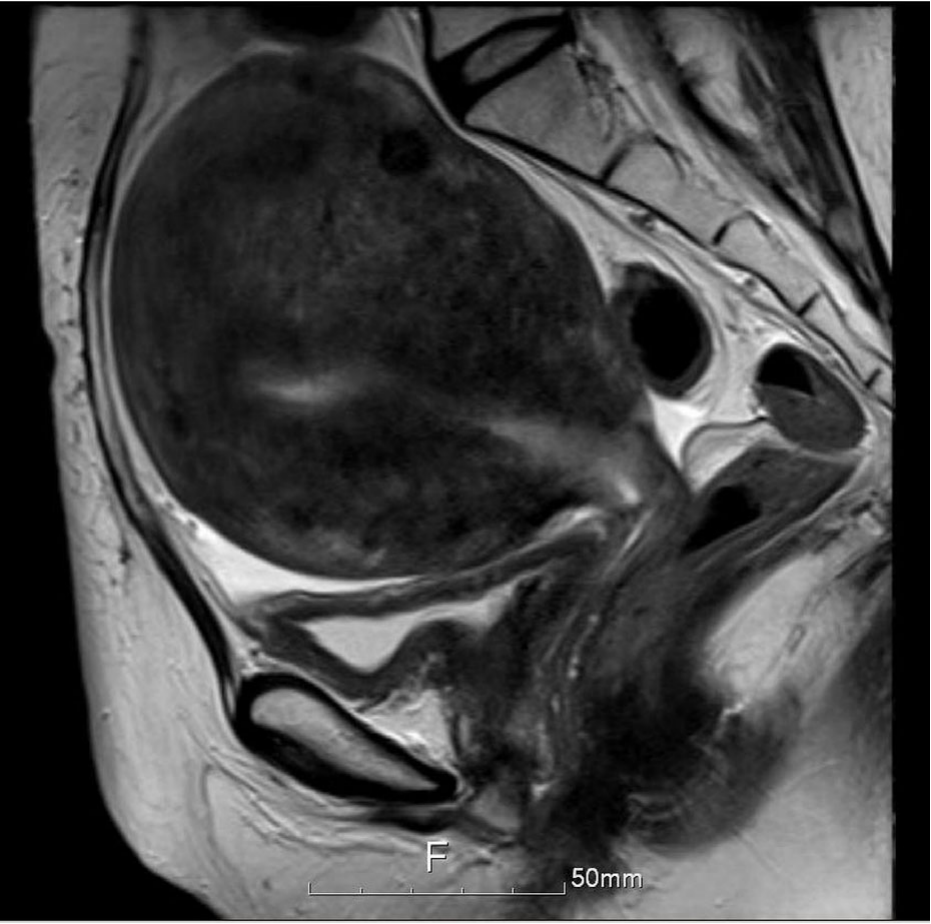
Pelvic MRI after concurrent chemoradiation therapy and HT-guided SBRT shows multiple uterine myomas and adenomyosis without local recurrence or pelvic lymphadenopathy.
